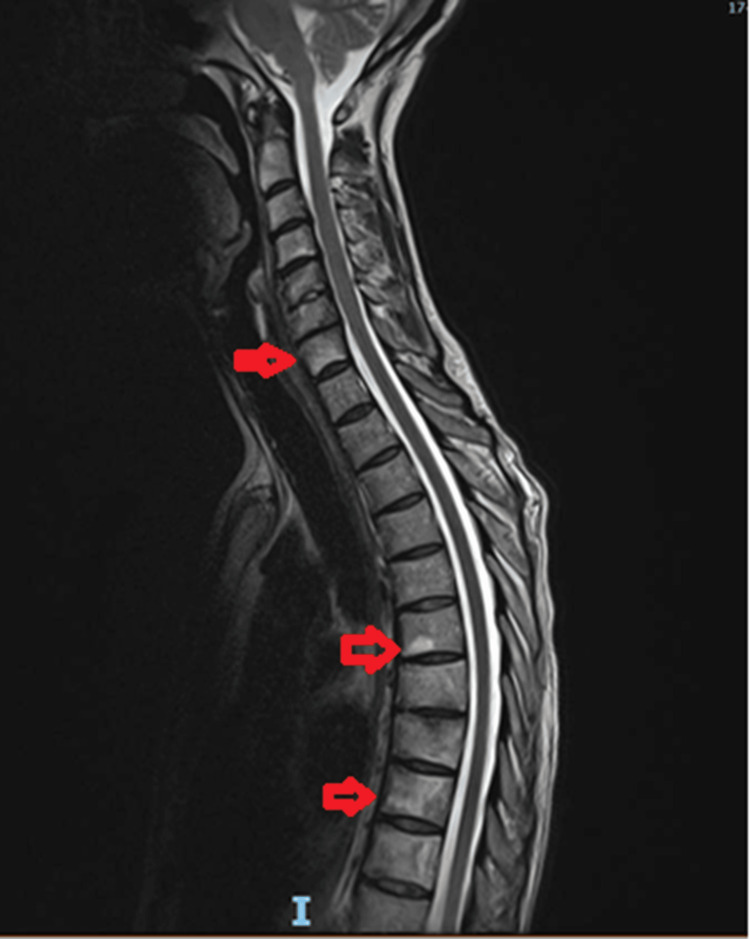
Sagittal T2W MRI image shows zones of vertebral bone marrow abnormality (sclerosis) extending from C5-C7 and T6-T10
